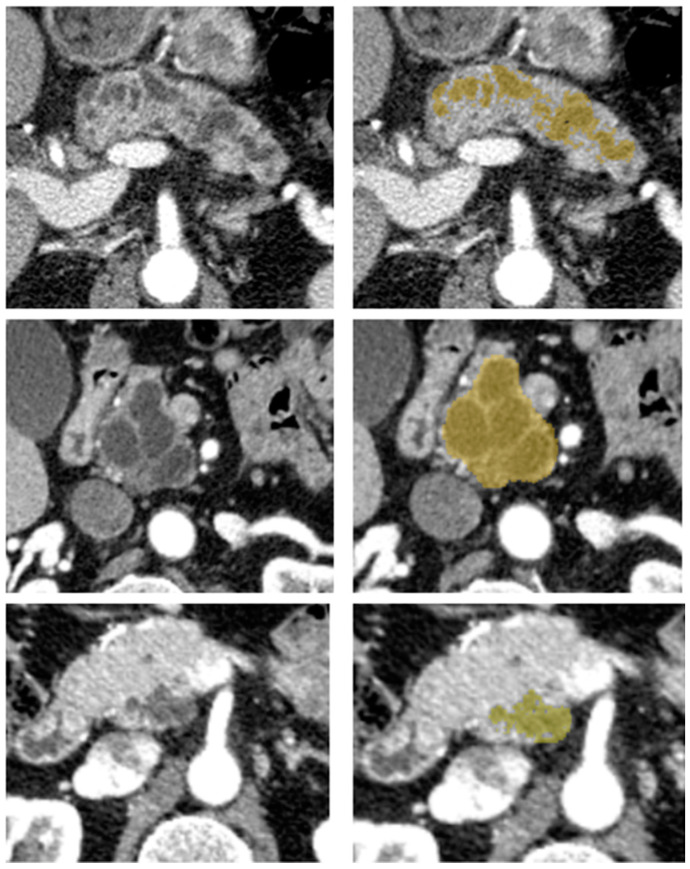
Examples of different IPMN on axial contrast-enhanced CT scans in the pancreatic phase (left) and with a semi-automatic outlined tumor in yellow (right).
